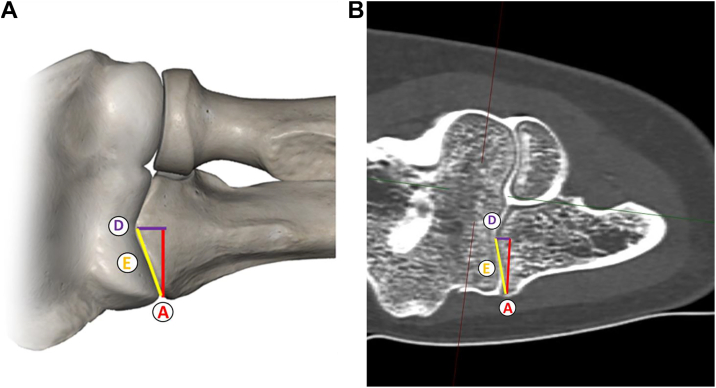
Graphical representation (A) and flexion computed tomography arthrography scan (B) of the assessments of proximal ulna and radius in coronal plane (mm): *A*, medial coronoid flange; *D*, maximum articular height at the coronoid midpoint; *E*, the hypotenuse between the segments A and D.
